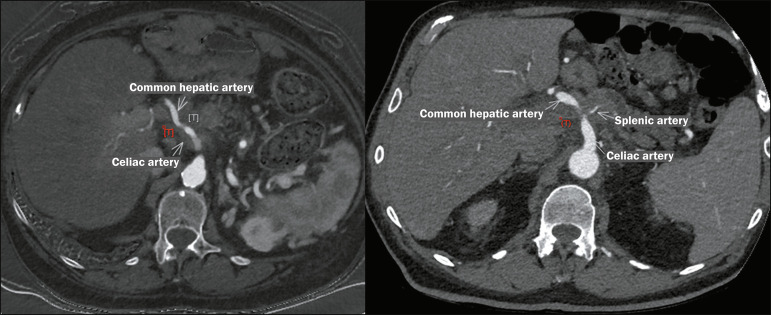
Axial contrast-enhanced CT scan of the abdomen, in a maximum
